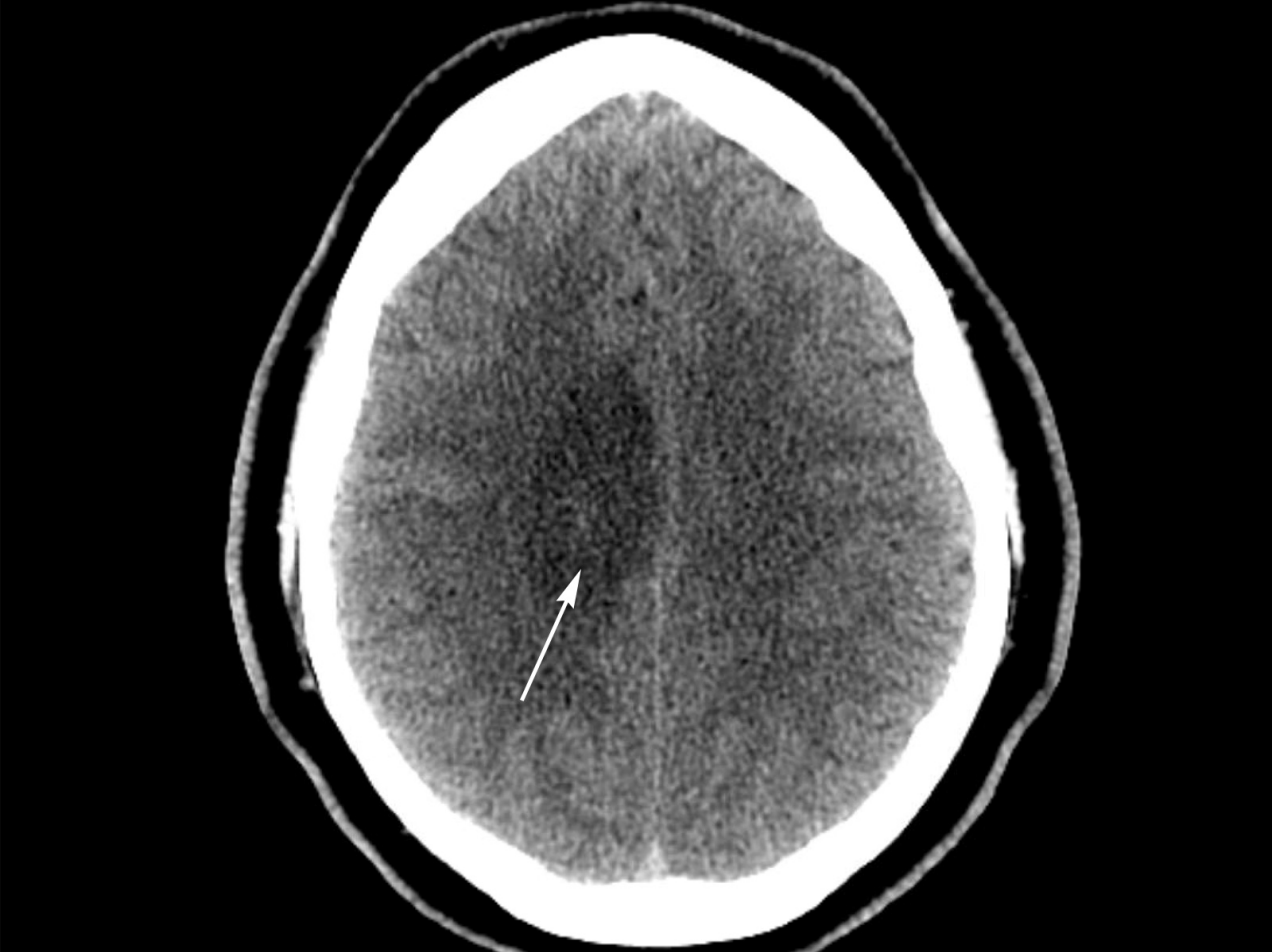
Non-contrast CT head demonstrating vasogenic edema surrounding underlying DVA
